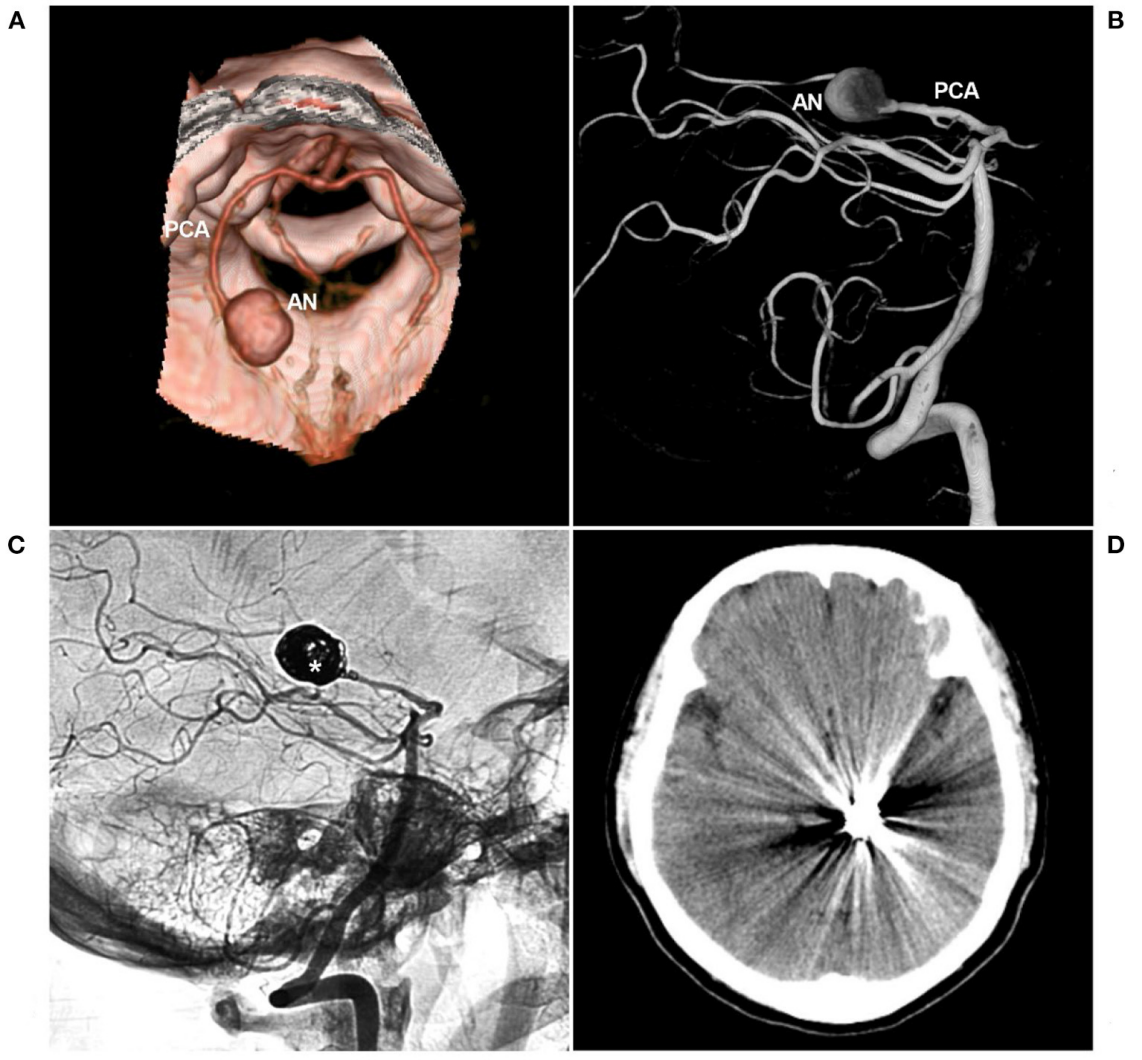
PAO for aneurysm of the P2-P3 junction. (A,B) CTA (A) and three-dimensional angiogram (B) showing the aneurysm (AN) on the left P2-P3 junction. (C) The aneurysm was treated by coiling PAO (asterisk). (D) Postoperative CT showing no infarction in the left occipital lobe. AN, aneurysm; CT, computed tomography; CTA, CT angiography; PAO, parent artery occlusion; PCA, posterior cerebellar artery.
